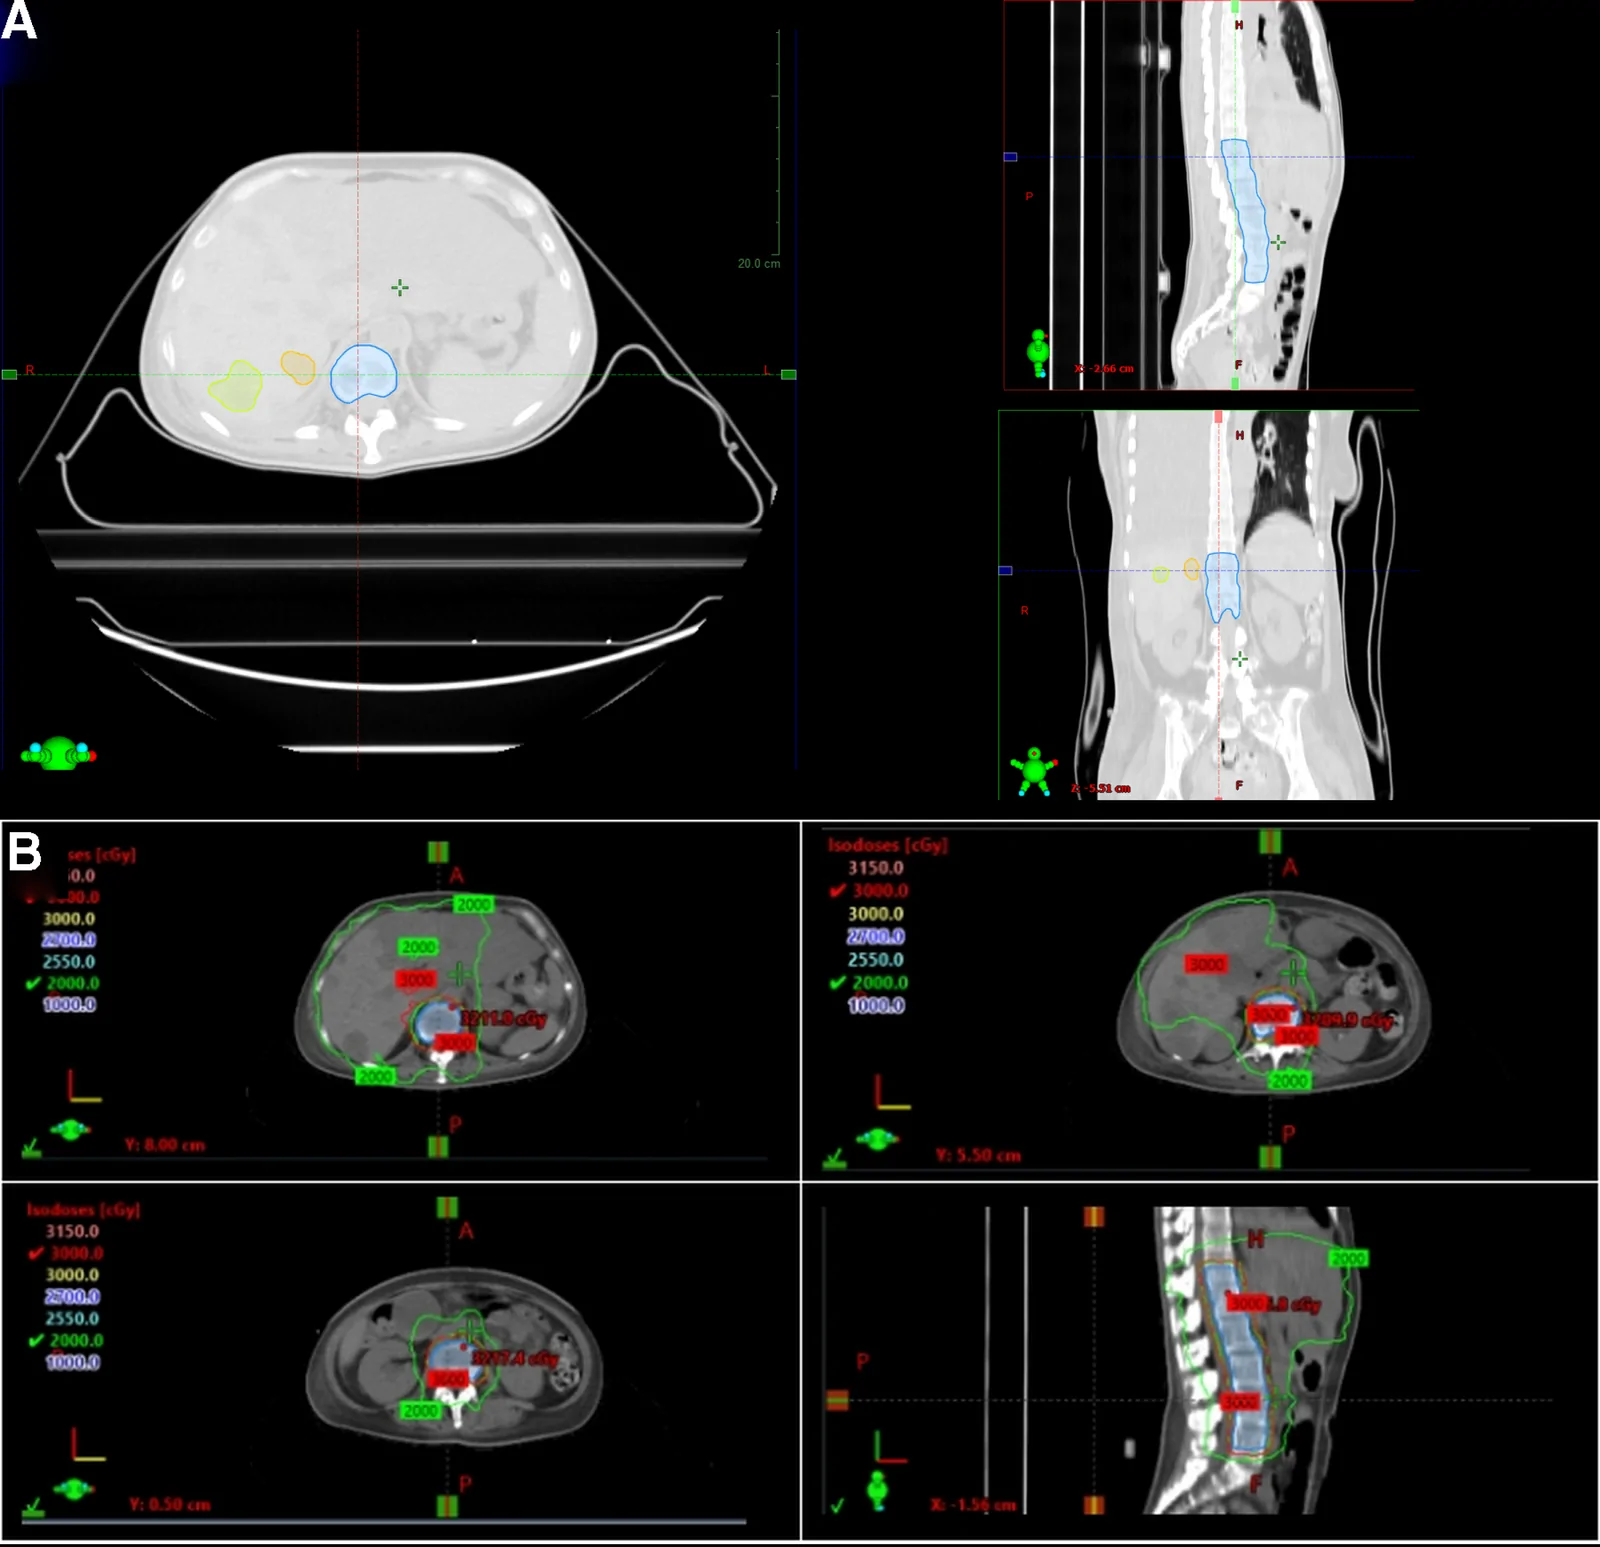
Target delineation and dose distribution for the third radiotherapy course. (A) Axial, sagittal, and coronal planning CT images demonstrate one lumbar metastatic target (blue contour) and 2 hepatic metastatic targets (green and yellow contours). (B) Representative axial and sagittal dose-distribution images demonstrate coverage of the 3 targets. All targets received 30 Gy in 10 fractions using a single VMAT plan. CT = computed tomography, VMAT = volumetric modulated arc therapy.
